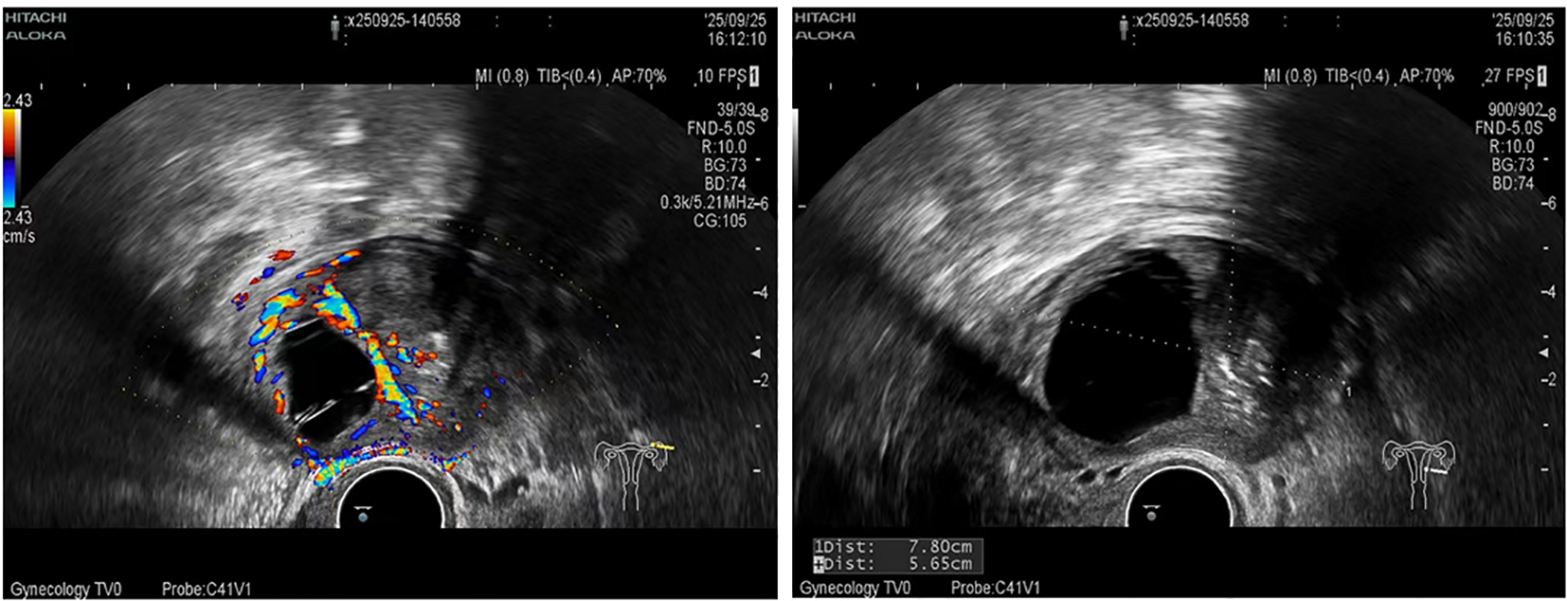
Ultrasound imaging demonstrating a mixed echogenic mass in the left adnexal region.
